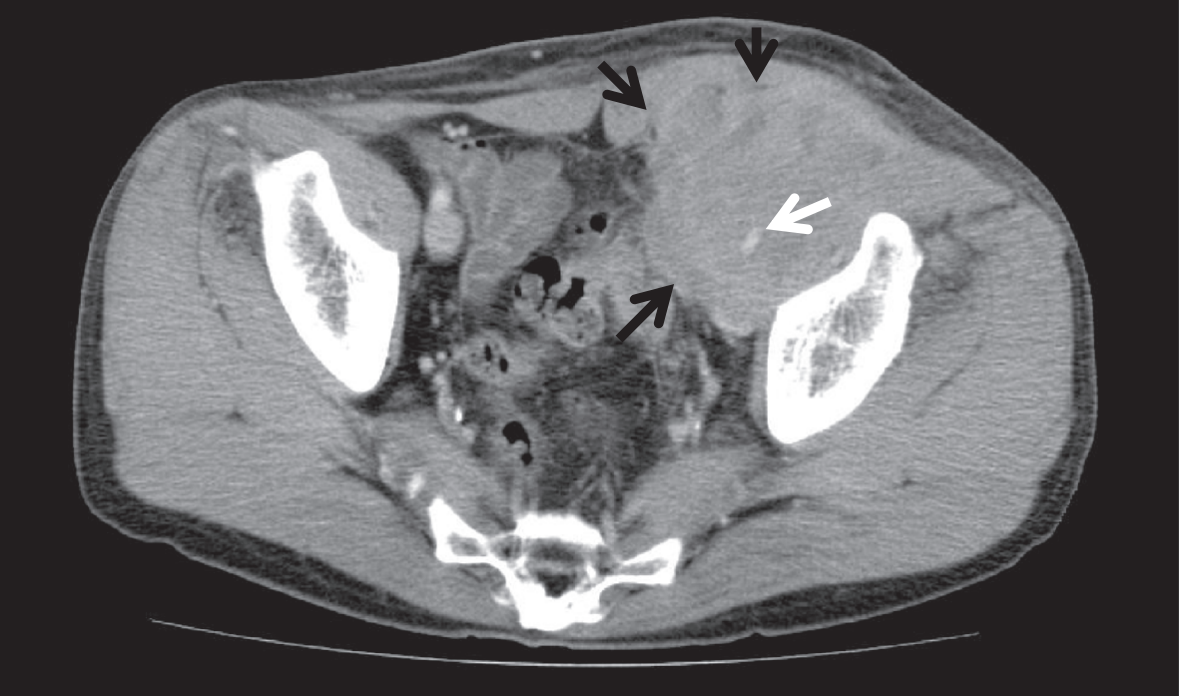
Abdominal contrast computed tomography revealed that a mass, approximately 7.0 cm in diameter (black arrows), was detected in the left inguinal region and the tumor involved the external iliac vein (white arrow).
